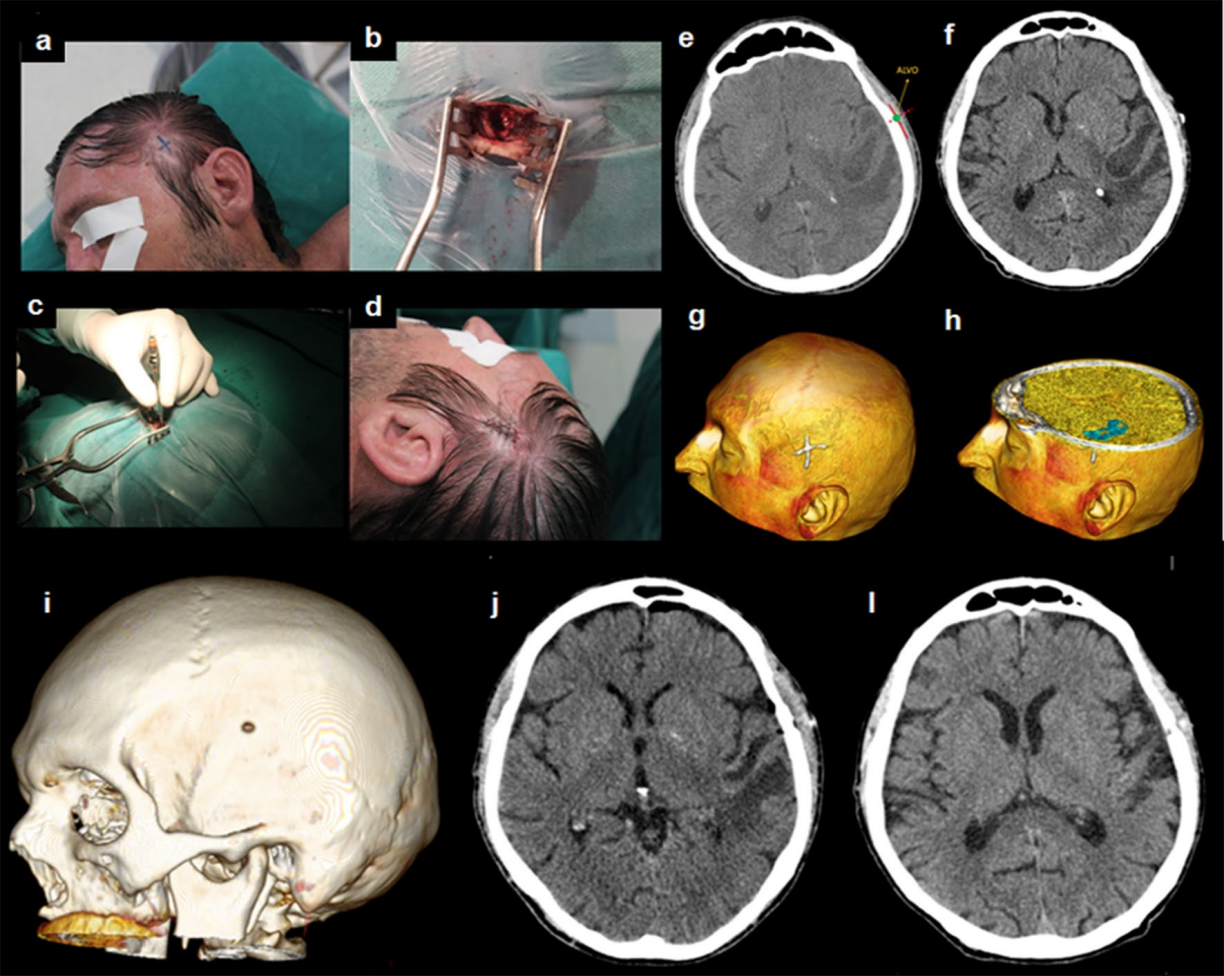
A 58-year-old man with a diagnostic hypothesis of left temporal brain abscess submitted to drainage through trepanation. (a), (b), (c), and (d) Intraoperative images showing the surgery step-by-step; (e) Surgical planning (f) Cranial CT scan after targeting; (g) and (h) pre-operative three-dimensional virtual reality reconstruction (3DVR) showing the target and its relationship with the lesion; (i) postoperative 3DVR with the trepanation; (j) Immediate postoperative cranial CT scan; (l) late radiological control, after 6 weeks, with complete resolution of the abscess.
